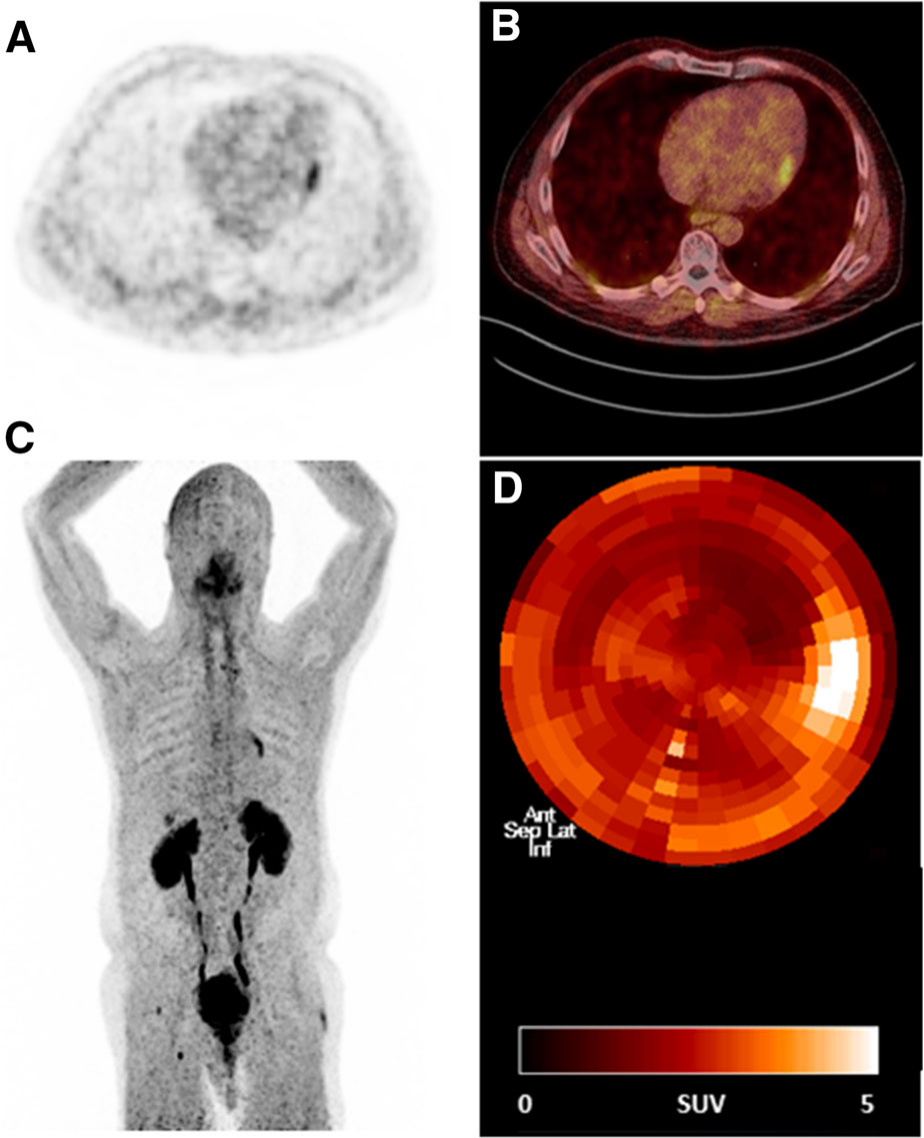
Localized lateral FAPI uptake in a patient (patient #22) without a known history of coronary artery disease. FAPI scan was performed for staging after treatment of urinary bladder carcinoma without evidence of recurrency but reactive FAPI uptake due to enthesopathic changes (both hips, right ischial tuberosity). (A) Trans-axial slice of Ga-68 FAPI PET, (B) fusion of trans-axial Ga-68 FAPI PET and low-dose CT, (C) maximum intensity projection of the whole-body PET, (D) polar map demonstrating spatial Ga-68 FAPI uptake in the left ventricular myocardium
